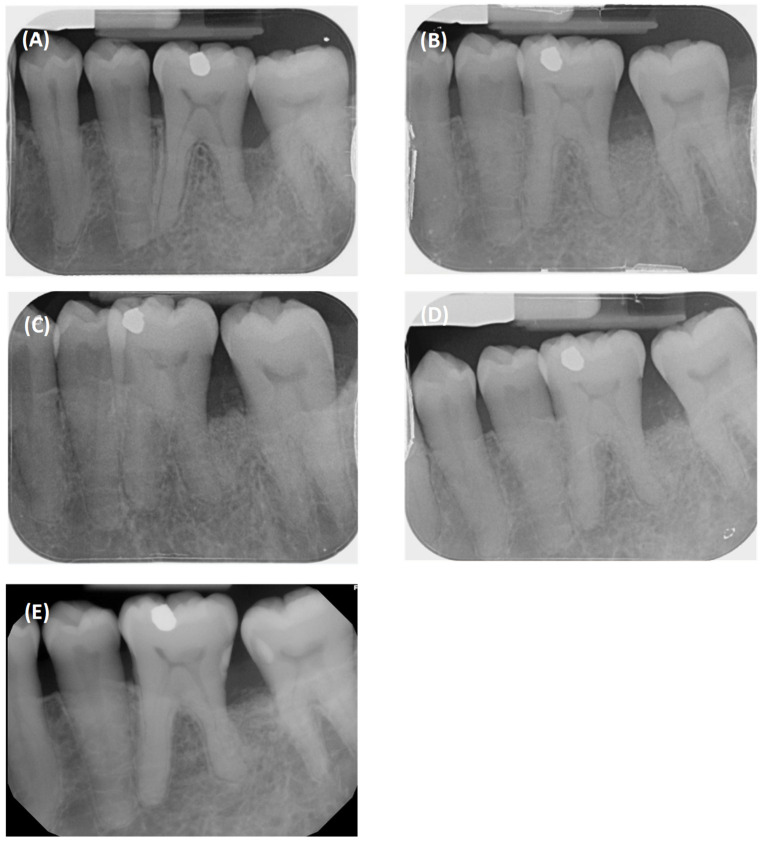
Radiographic follow-up of #36: (A) pre-op; (B) 6 months; (C) 1 year; (D) 1.5 years; (E) 3 years.
